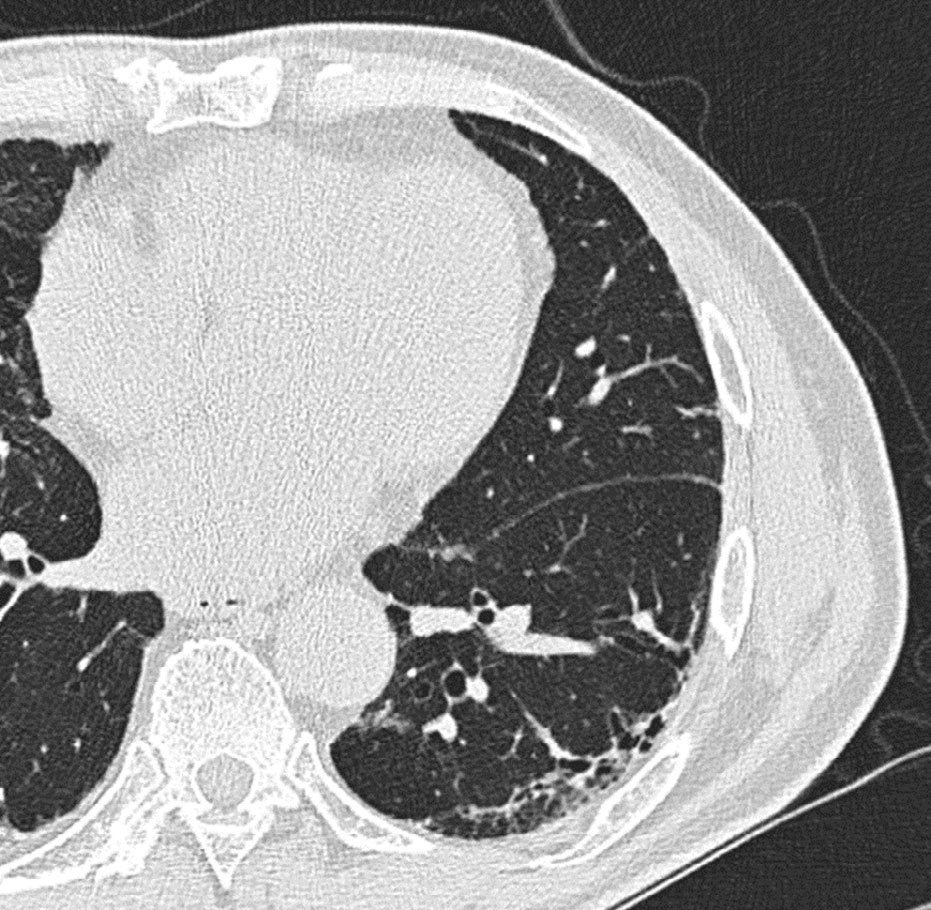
High‐resolution computed tomography image showing a typical example of pre‐existing interstitial lung disease (probable usual interstitial pneumonia pattern)
